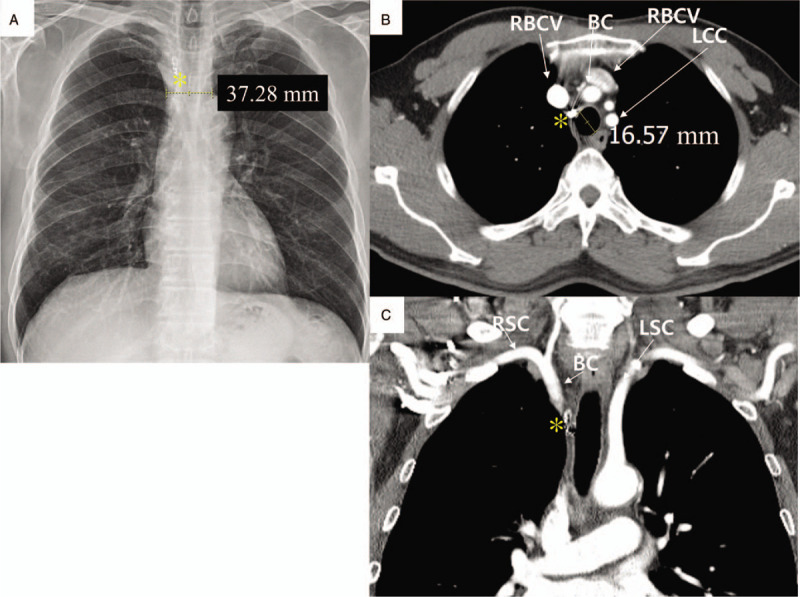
Post-discharge follow-up study. A. Chest X-ray image at 1-month post-discharge shows the normalization of mediastinal widening. The number indicates the mediastinal size just above the aortic arch level. B. Chest computed tomography image at 2 months post-discharge shows the recovery to the normal range of mediastinal and trachea, except for remnants of embolization therapy. The numbers indicate the tracheal diameters. C. Chest computed tomography coronal section image at 2 months post-discharge shows the occluded terminal branch of the right inferior thyroid artery, with endovascular embolization and complete resolution of the mediastinal hematoma. An asterisk indicates the Tornado microcoils and glue remnants applied to the bleeding vessels. BC = brachiocephalic artery, LBCV = left brachiocephalic vein, LCC = left common carotid artery, LSC = left subclavian artery, RBCV = right brachiocephalic vein, RCS = right subclavian artery.
